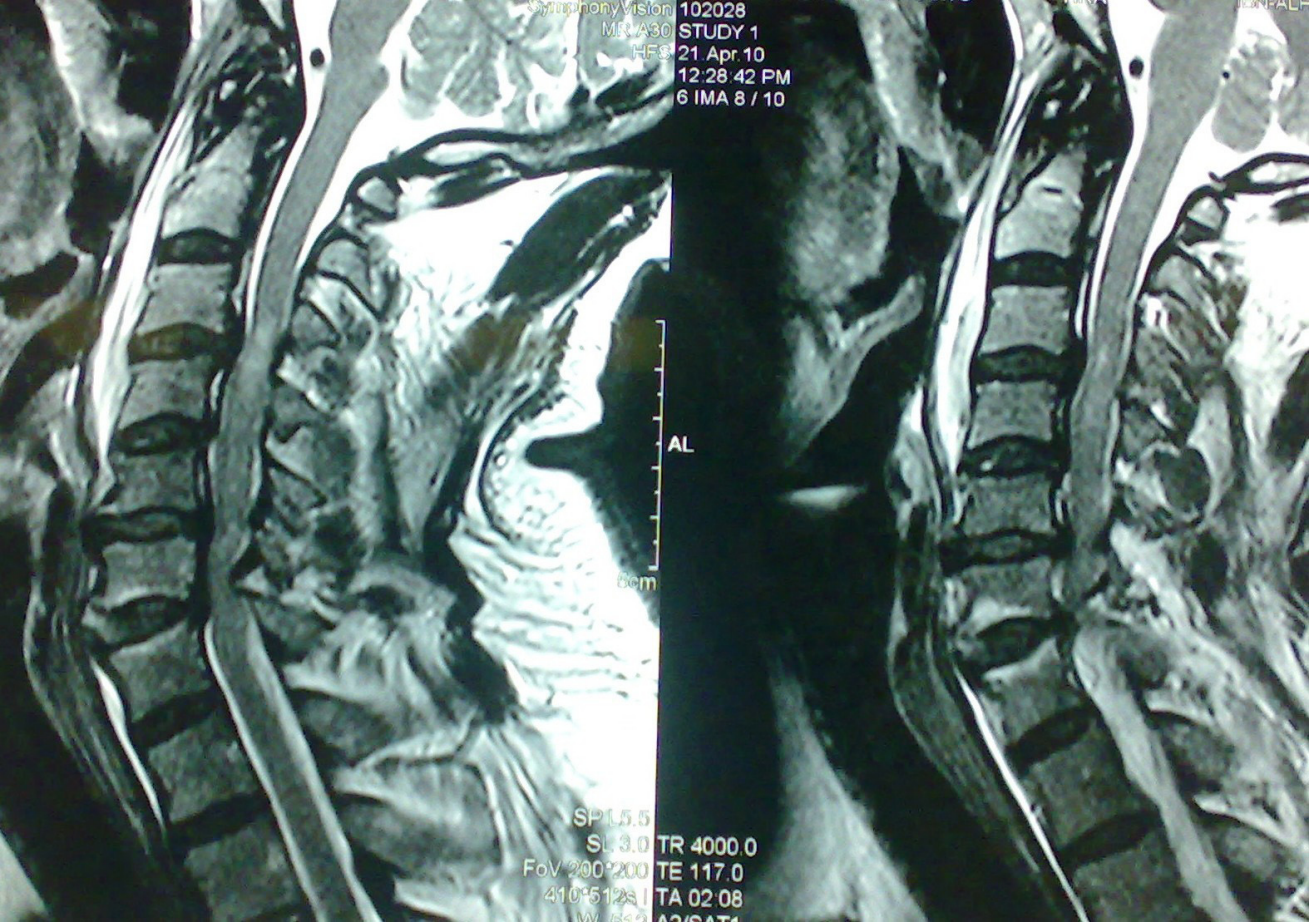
Pre operative sagittal MRI T2W. Reveals severe cervical spine spondylotic changes with a spinal cord signal at C3/4 level in a patient of a 65 years old with traumatic spinal cord injury.
